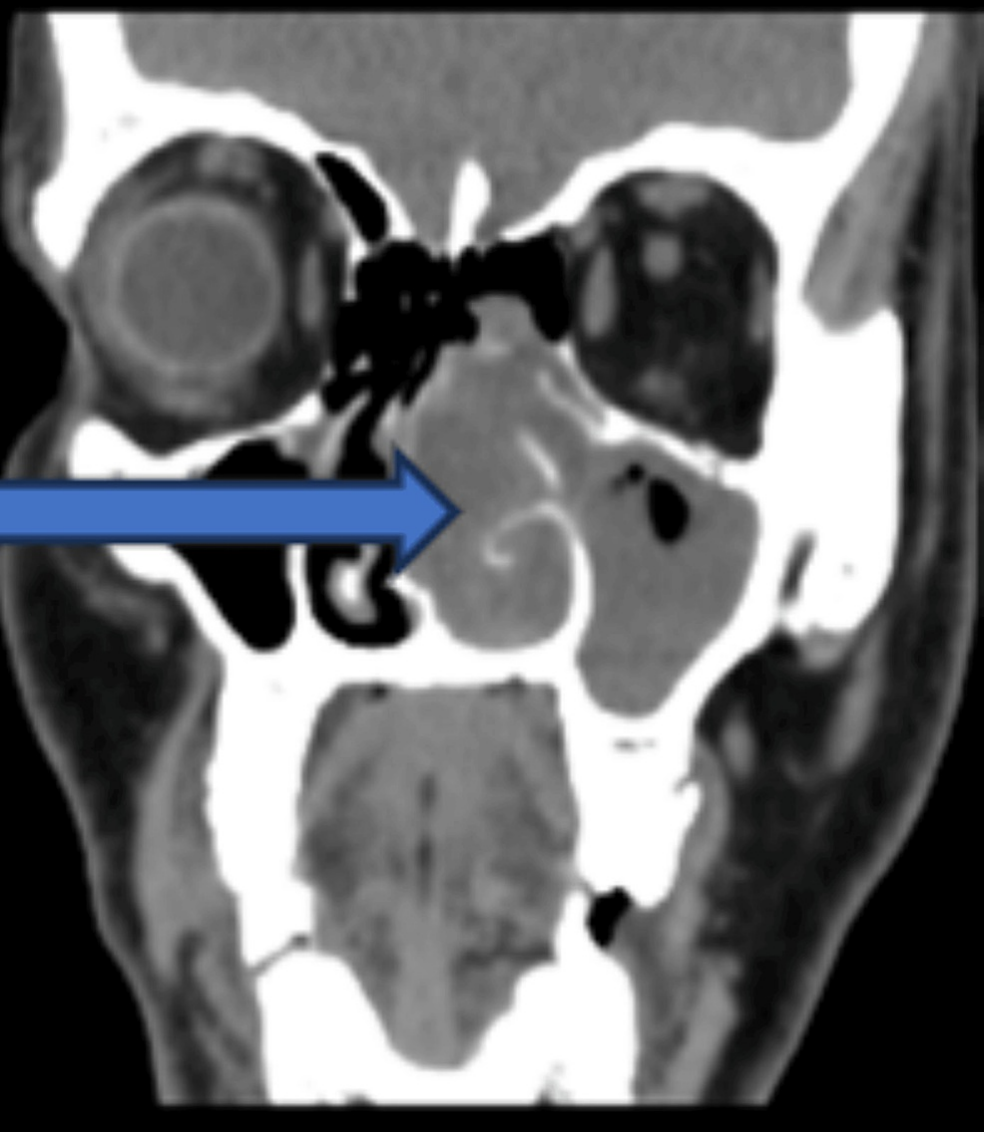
CT scan suggestive of unilateral isodense lesion suggestive of inverted papilloma (solid arrow)
